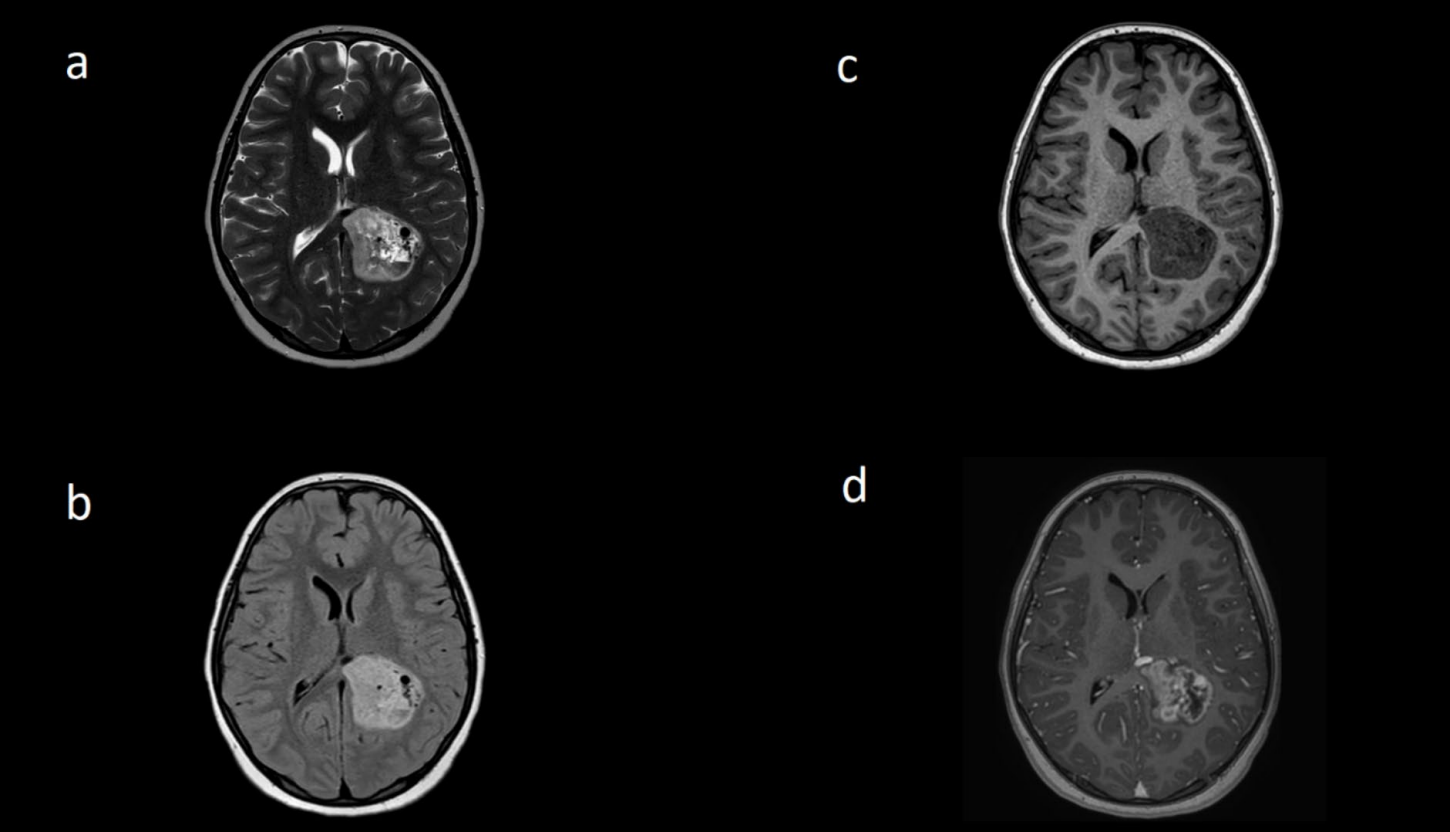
Preoperative axial MRI sequences: a T2, b FLAIR, c T1 and d T1 + gadolinium
